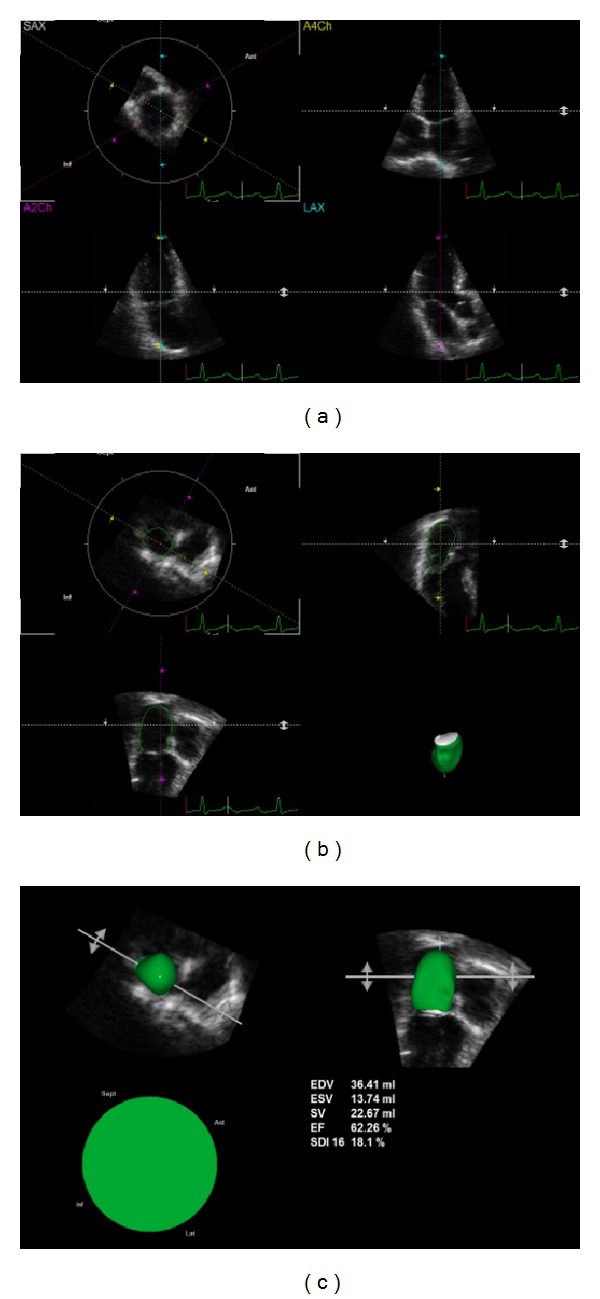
Left atrial volumes by TomTec 4D echocardiography. (a) After LA full-volume data acquisition, TomTec software displays LA in 3 different apical planes (4-chamber; 2-chamber, long-axis). (b) The endocardial border is surrounded on end-diastolic and end-systolic frames and, if needed, manually corrected. (c) Atrial maximum (max) and minimum (min) volumes are obtained using semiautomated border detection algorithm and displayed in numeric values and in 3D image (in green).
